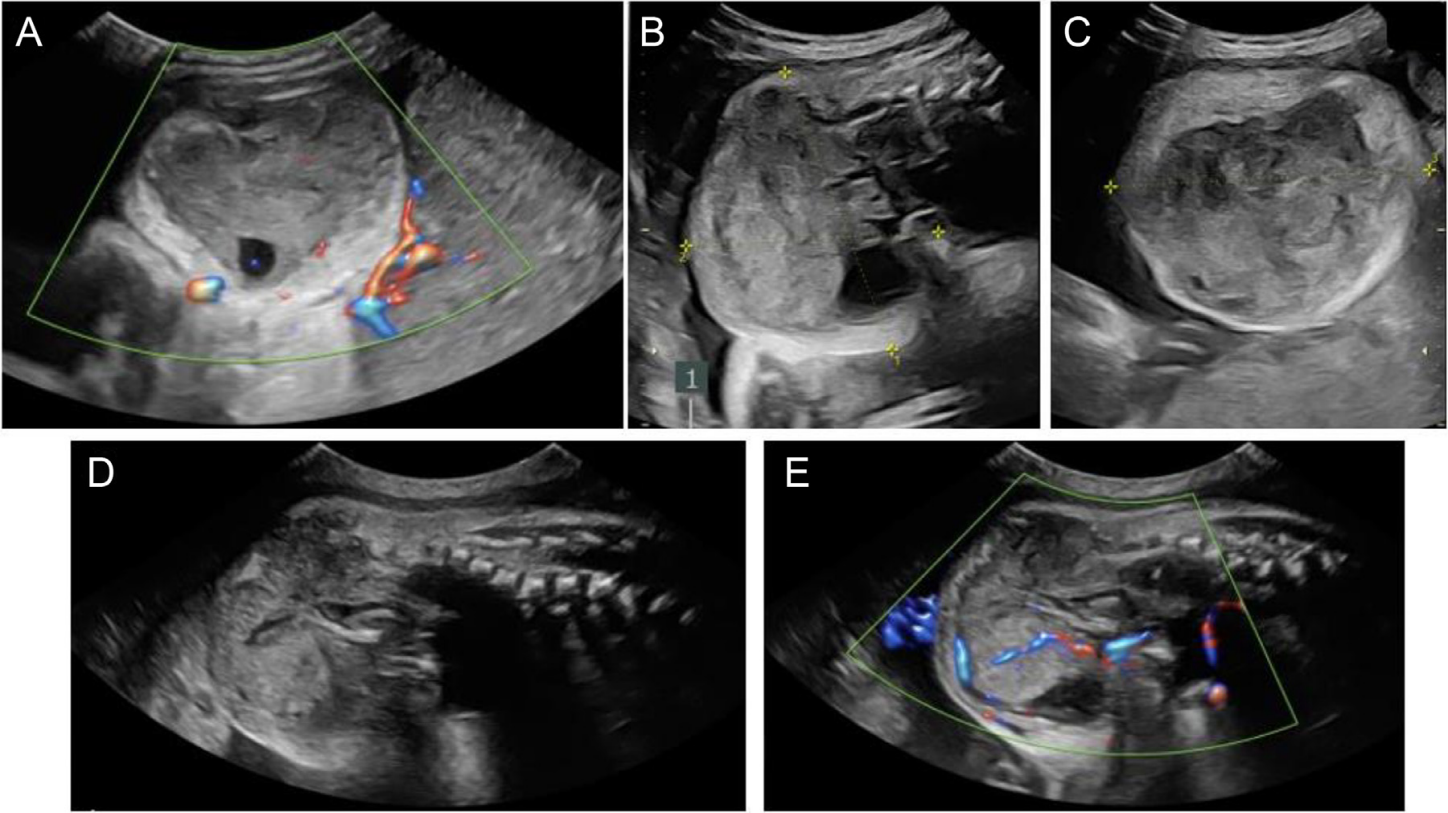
Fetal ultrasound performed at 34 weeks 6 days gestation. The transverse (A-C) and sagittal (D, E) planes demonstrate a mass exophytic to the distal spine. The mass can be seen extending from the distal aspect of the spine measuring 7.63 × 6.47 × 8.17 cm (B, C). Two-dimensional color mapping (A, E) demonstrates the vascular nature of the mass.
